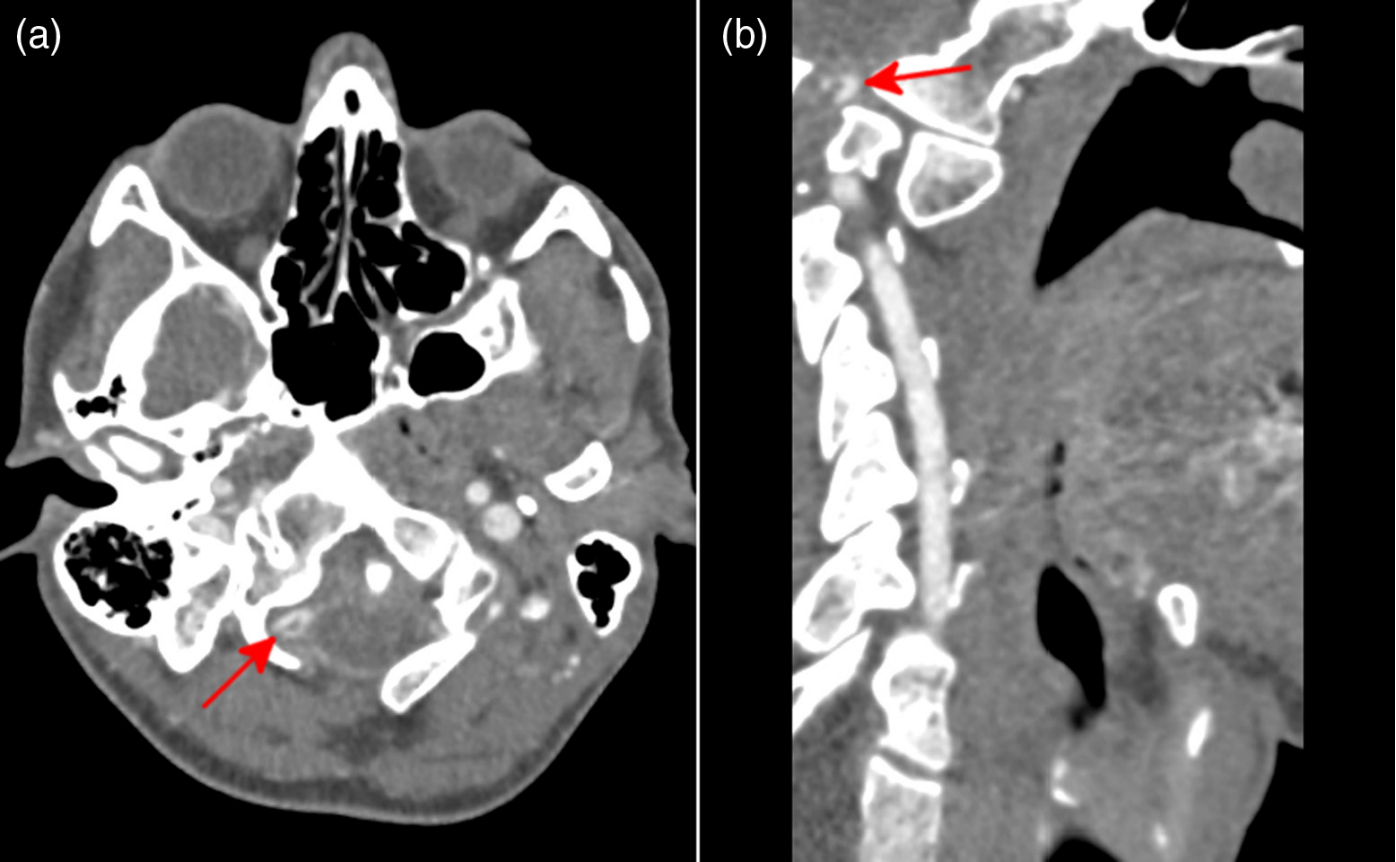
Computed tomography angiography of the head and neck showing short segment intimal dissection in the right vertebral artery just above the level of the foramen magnum (arrows) on (a) axial and (b) oblique views.
